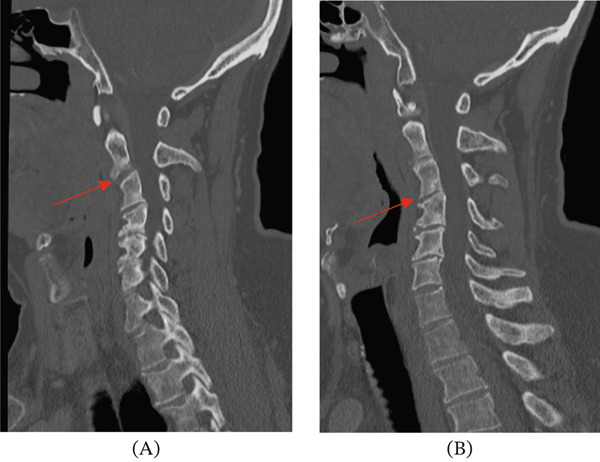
These CT scans of the cervical spine in our patient show that the (A) right C2–C3 uncovertebral joint (red arrow) and (B) left C3–C4 uncovertebral joint (red arrow) lesions are poorly visualized on CT, showing no significant sclerotic or lytic features.
